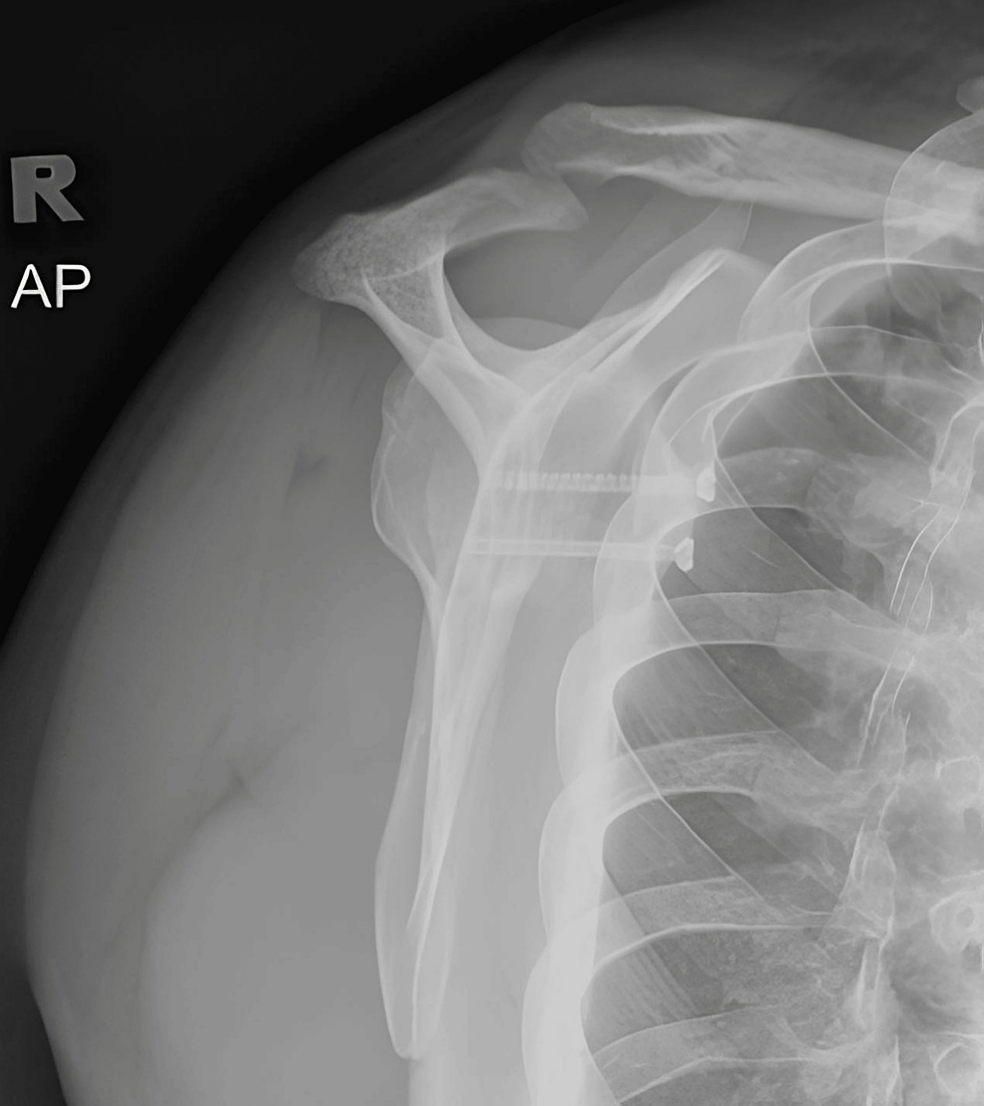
Postoperative radiograph (scapular Y view) showing right shoulder Latarjet procedure.
